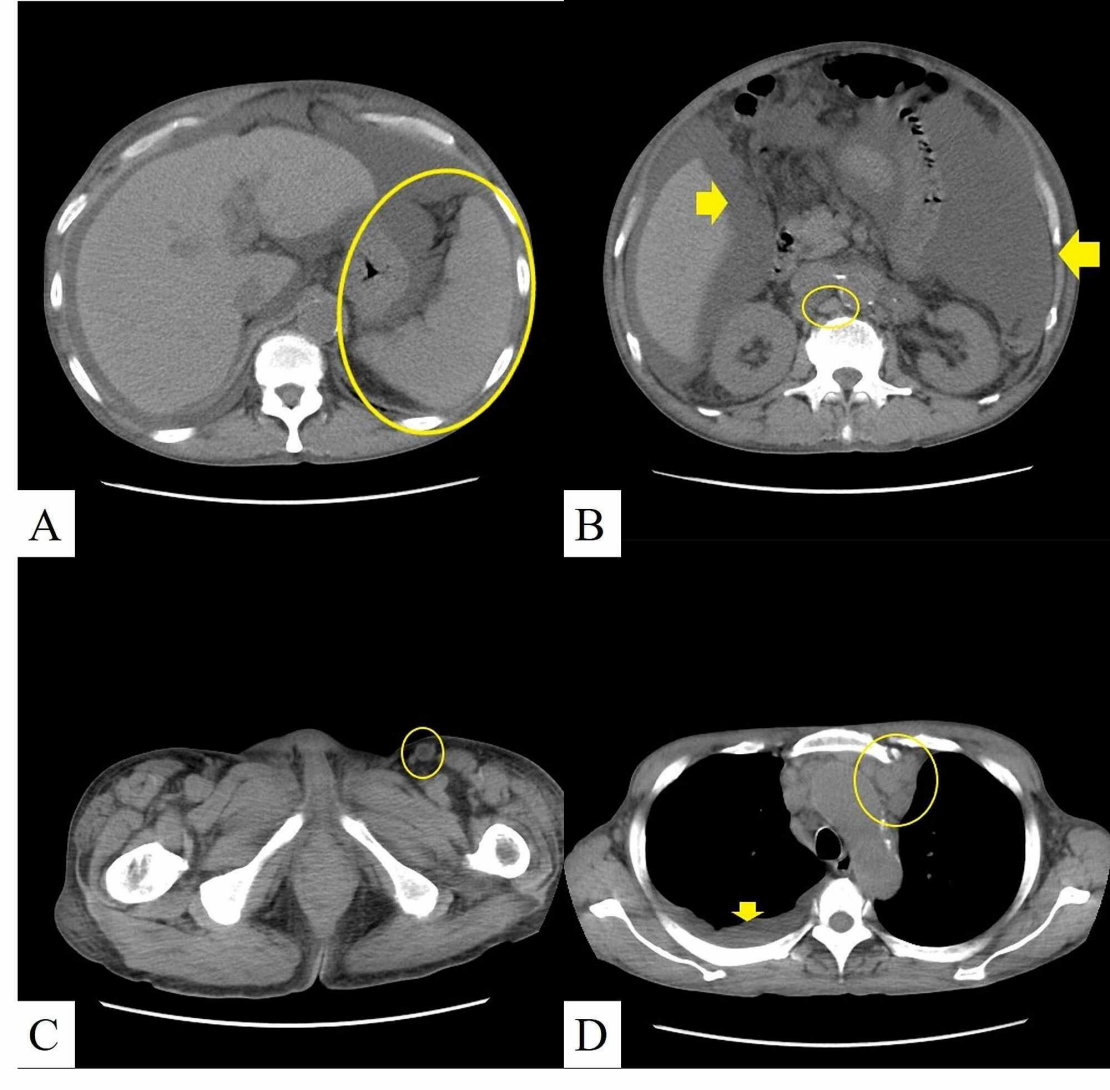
A computed tomography scan revealed splenomegaly (circle) (A); massive ascites (arrows); mildly enlarged lymph nodes in the para-aorta (circle) (B); inguinal (circle) (C), and mediastinal regions (circle); and pleural effusion (arrow) (D)
